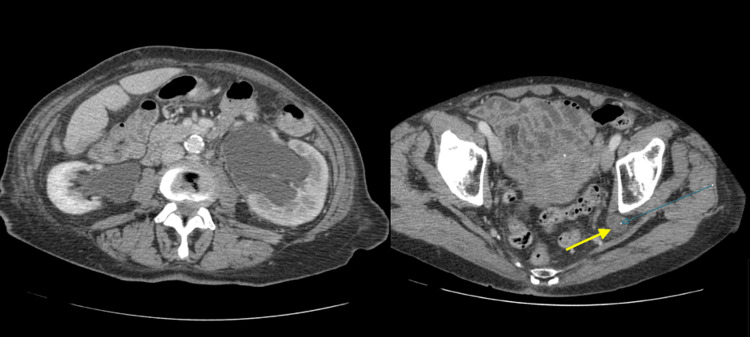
CT scan showing severe hydronephrosis on the left side with herniation of the left ureter through the great sciatic foramen (arrowed).
